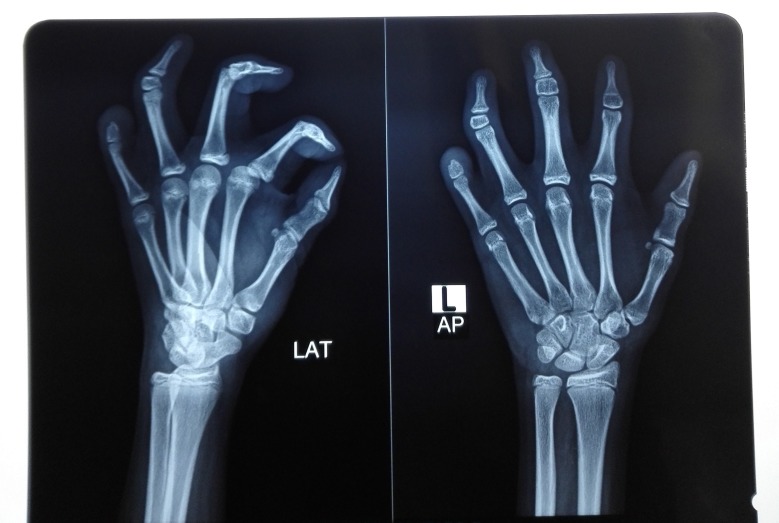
X-ray images of the skeletal abnormalities
